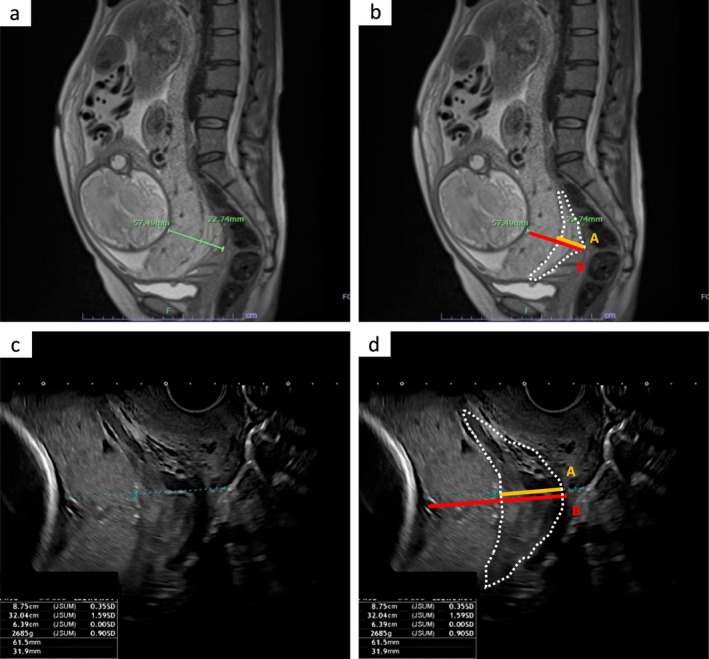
Imaging of cervical hypervascularity during pregnancy: TVUS and MRI findings. These images include sagittal T2‐weighted MRI scans (a and b) obtained at 33 weeks of gestation and transvaginal ultrasonographic images (c and d) acquired at 35 weeks in a patient with placenta previa. The region outlined by the white dashed line in panels (b) and (d) represents the cervical varicosity, which was defined based on its characteristic vascular morphology along the cervical canal. The positional relationship between the cervical varicosity, the placenta, and the serosal surface is indicated as A/B.
